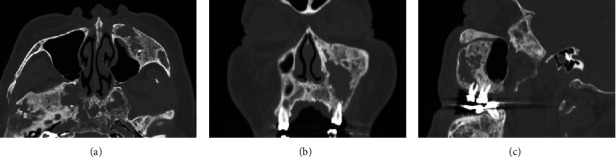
Computed tomography (CT) image obtained at the initial visit. CT shows bone hyperplasia and “ground-glass” appearance at the left maxilla, mandible, and sphenoidal bone, along with an osteolytic lesion extending from the root apex of the left maxillary first molar to the inferior aspect of the left infraorbital foramen and canal in the “ground-glass” lesion. Axial (a), coronal (b), and sagittal (c) CT views.
